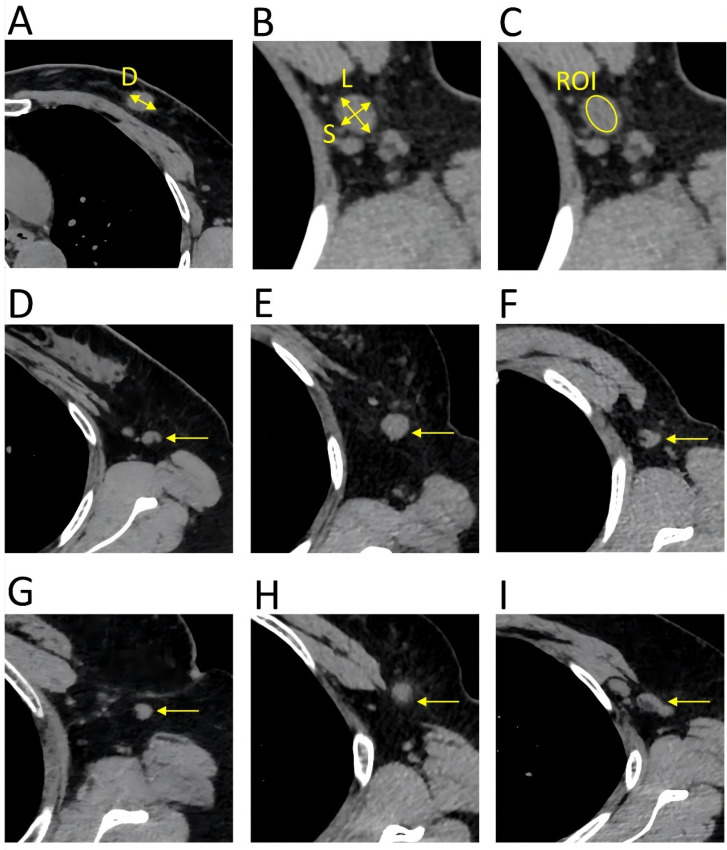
Key parameters of the HRCT imaging. The primary tumor and lymph nodes were evaluated in the transverse of the HRCT imaging, with a slice thickness of 1 mm. A. (D) diameter of the primary tumor. B. (L) long diameter of the lymph node; (S) short diameter of the lymph node. C. The mean CT value was calculated by delineating a region of interest that encompassed the entire lymph node, ensuring that the measurement line remained within the border of the lymph node. D. Reniform shape. E. Round shape. F. Other shape. G. Smooth border. H. Indistinct border. I. Fused border.
